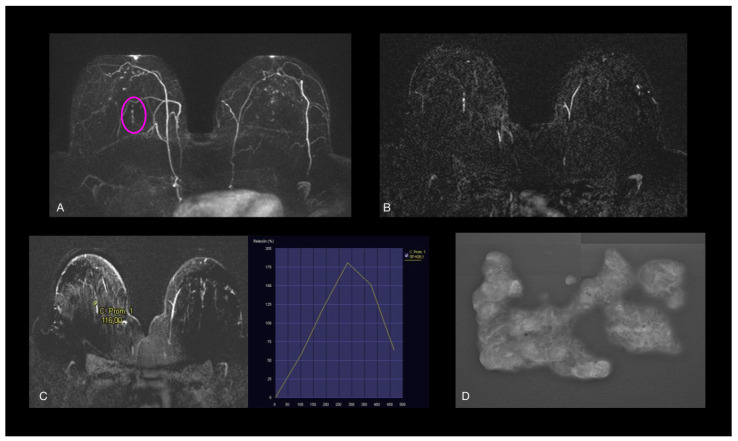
(A,B) Axial early postcontrast T1-weighted MR images show linear enhancement (pink oval), with (C) type 3 dynamic curve, which measured 20 mm in long-axis diameter. No US features. (D) Stereotactic biopsy revealed low-grade nuclear DCIS with necrosis.
